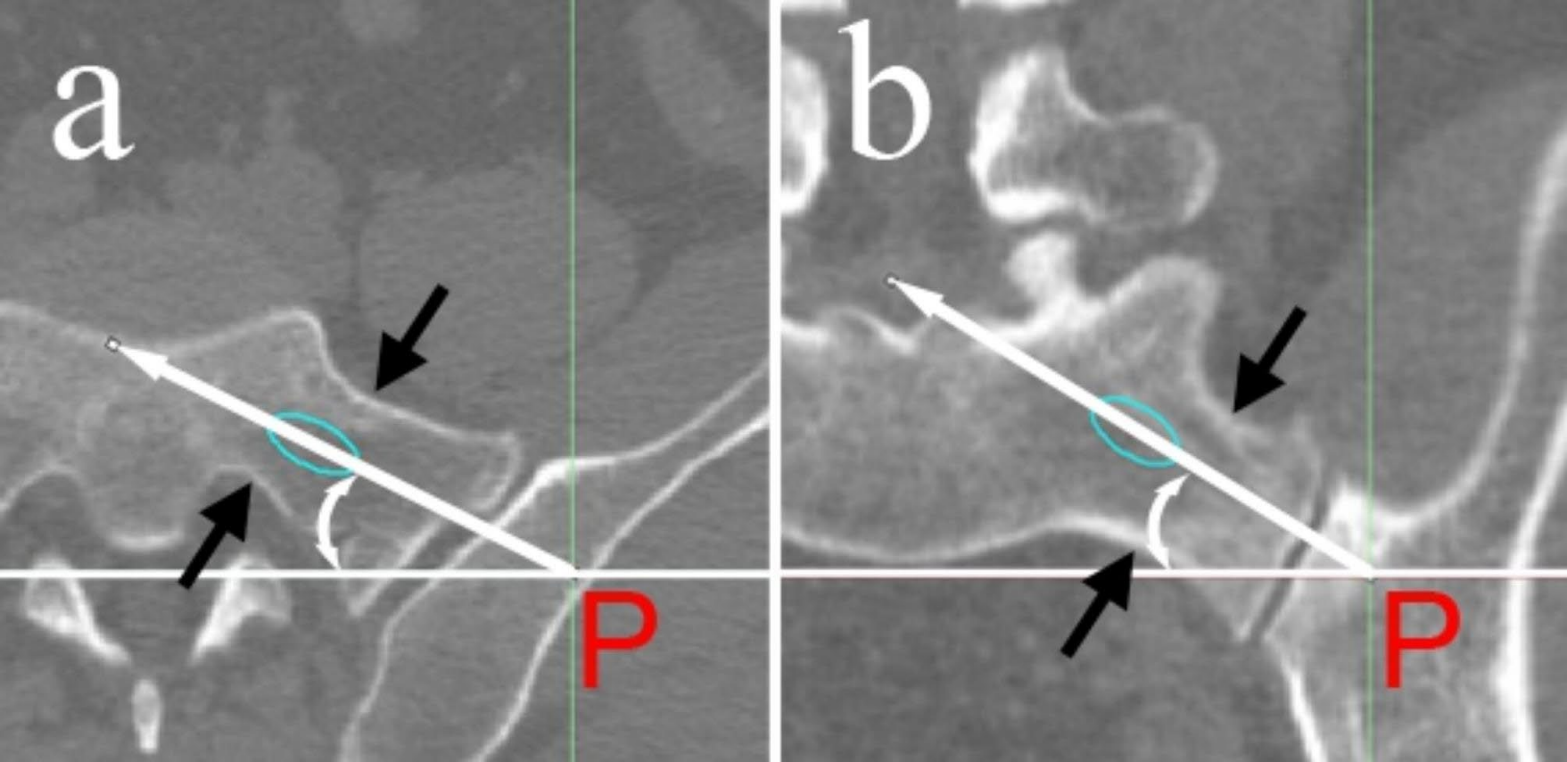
Measurement of the inclination angles of the upper SI screw. The green ellipse represented the screenshot of the screw on the CT scan, and the long white arrows represented its axis directions. (a) On the axial scan, the two short black arrows pointed out the nearest anterior and posterior cortical bone to the screw. (b) On the coronal scan, the two black arrows pointed out the nearest superior and inferior cortical bone to the screw
